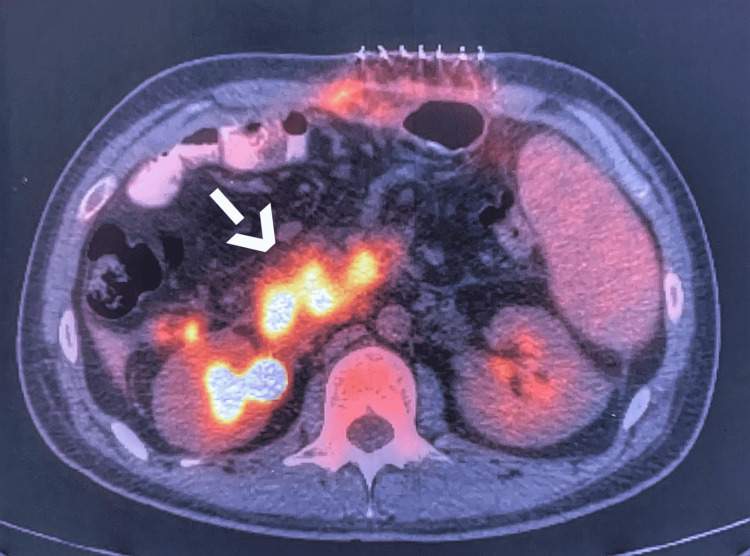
PET-CT image showing the pancreatic tumor (white arrow)
